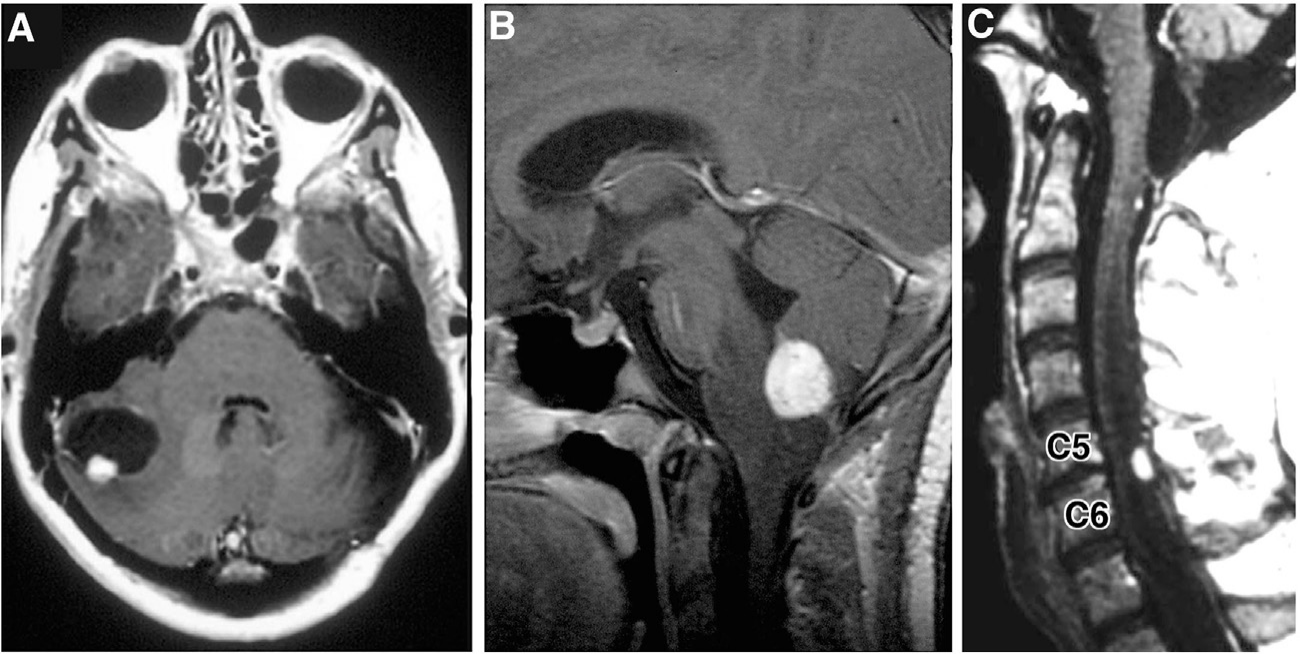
Radiographic images of hemangioblastomas. (A) Axial, contrasted, T1-weighted MRI showing cerebellar hemangiolastoma with contrast enhancing mural nodule and peritumoral cyst. (B) Sagittal, contrasted, T1-weighted MRI revealing contrast enhancing medullary hemangioblastoma with surrounding vasogenic edema. (C) Sagittal, contrasted, T1-weighted MRI with contrast enhancing posterior/dorsal hemangioblastoma with associated syrinx [adapted from Lonser et al. (7)].
